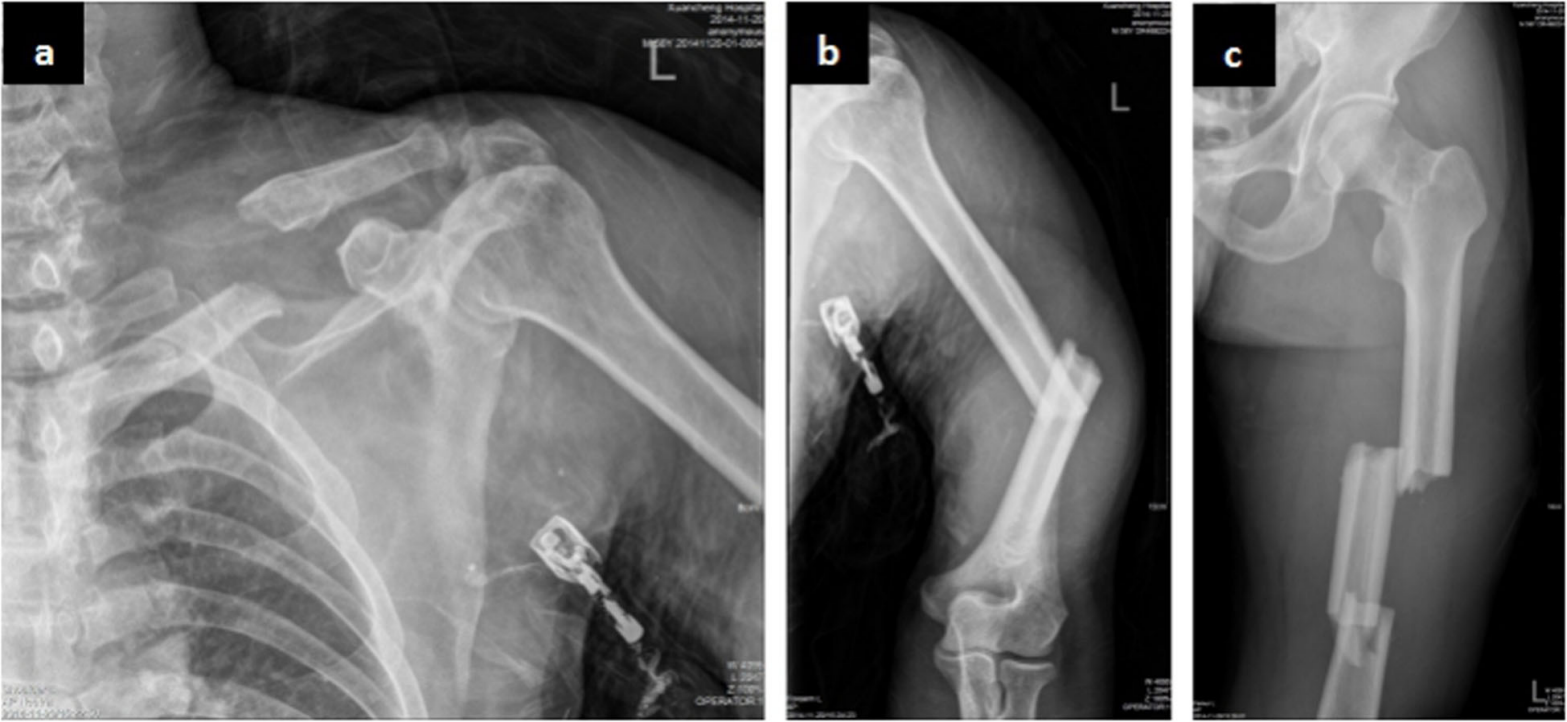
Radiographical assessment of multiple fracture, fractures of left clavicle (a), humeral shaft (b), femoral neck and femoral shaft (c)
